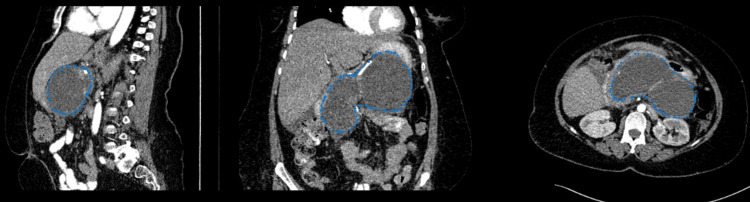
The first case CT scan showing the WOPN in three different planes (marked in blue). CT: computed tomography; WOPN: walled-off pancreatic necrosis
